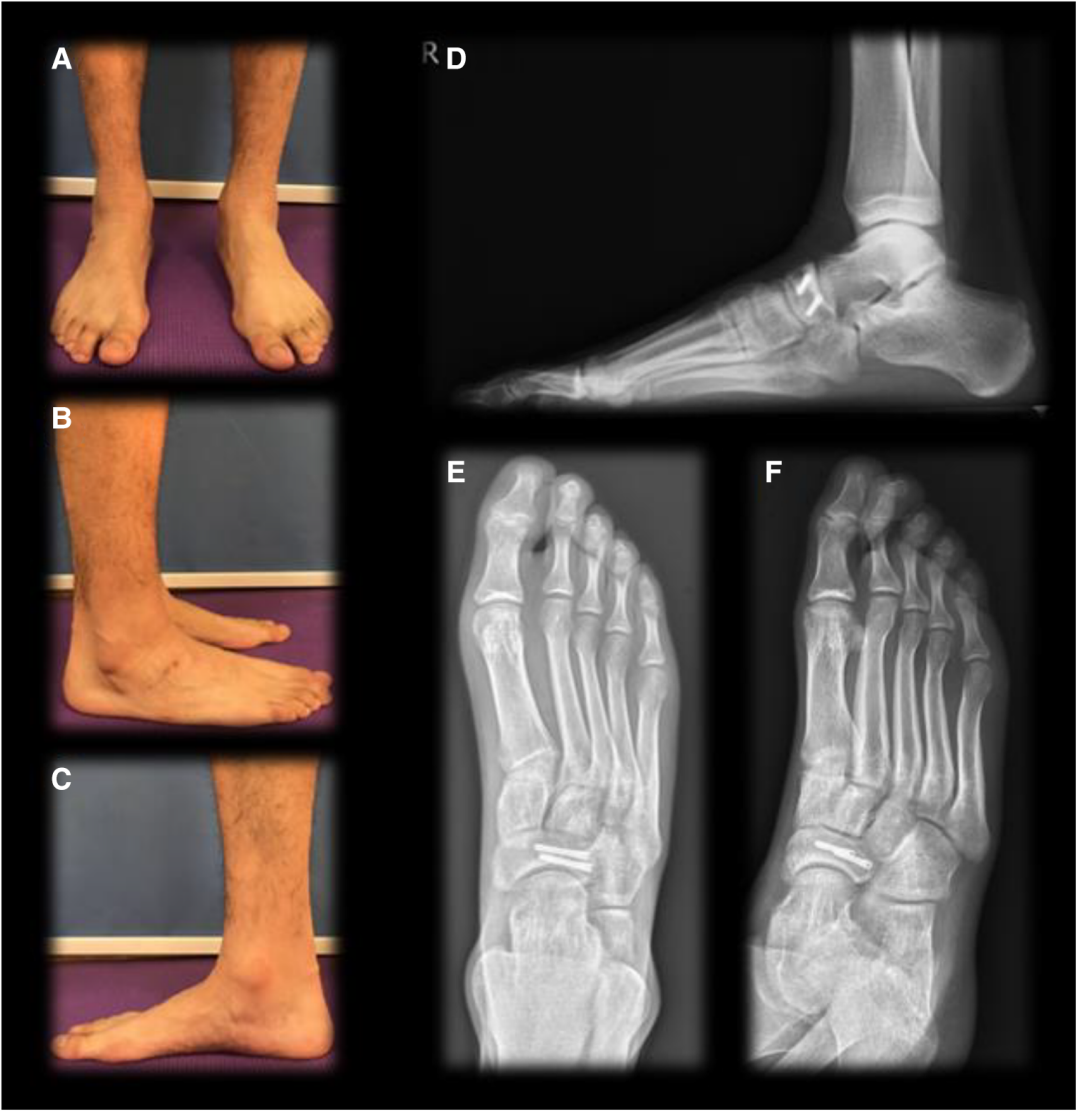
Twelve weeks postoperative follow-up. (A) Front view standing on two feet; (B) lateral view of the affected foot standing on two feet; (C) medial view of the affected foot standing on one foot; (D) weight-bearing lateral x-ray of the right foot; (E) anteroposternal x-ray of the right foot; (F) oblique x-ray of the right foot.
